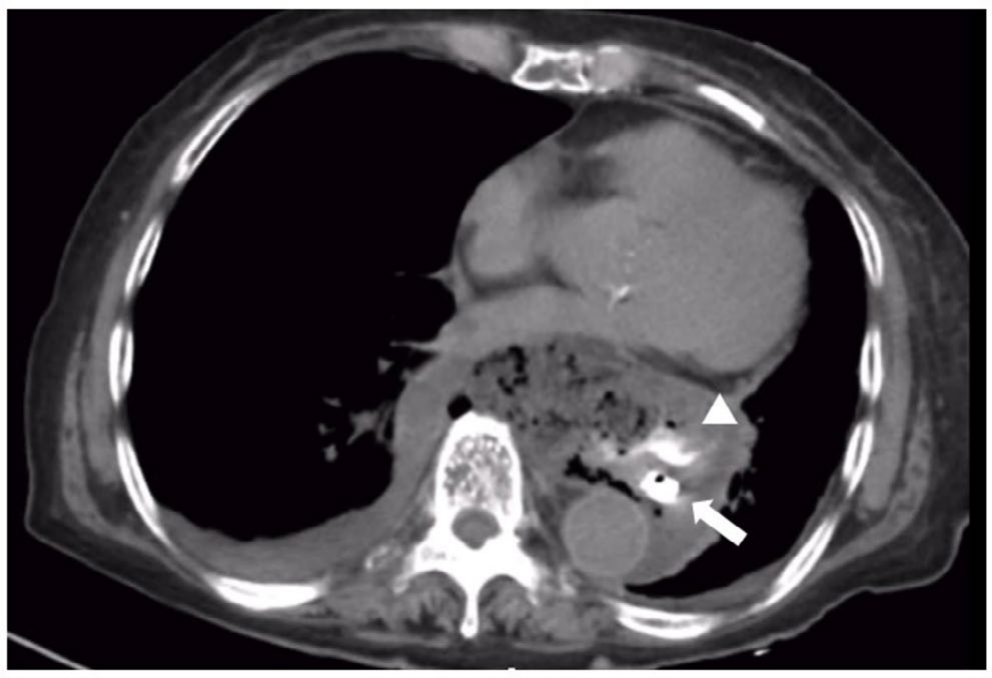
Preoperative computed tomography after upper gastrointestinal contrast study. Contrast extravasation was observed from the anterior‐right mediastinum of the lower esophagus, suggesting lower esophageal perforation (white arrowhead: Contrast extravasation; white arrow: Intraluminal contrast and nasogastric tube).
